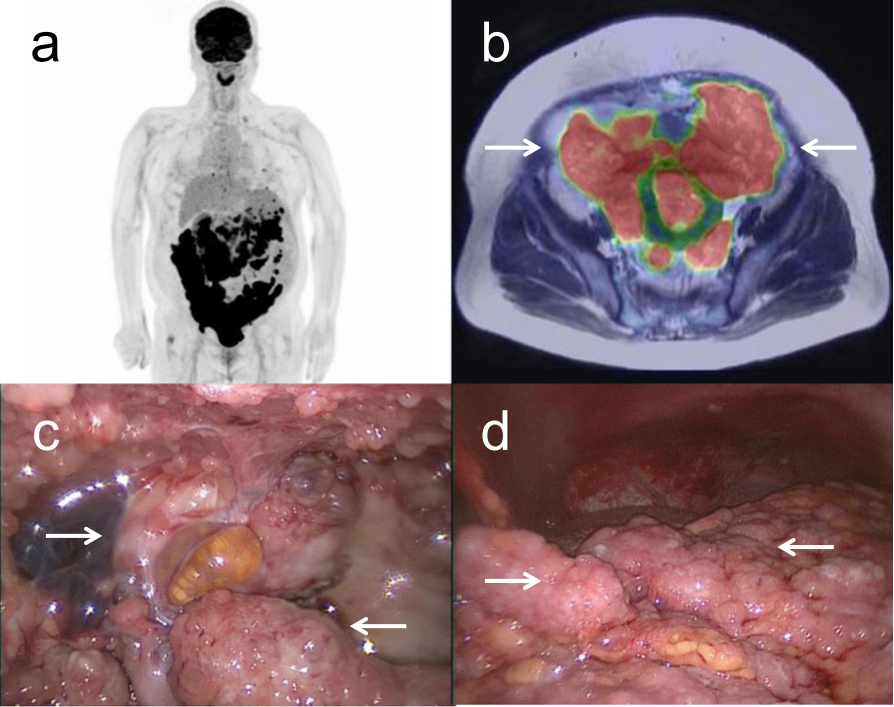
Whole-body FDG PET shows strong FDG uptake in multiple peritoneal nodule lesions (a). Integrated FDG-PET/T2-weighted MRI shows strong FDG uptake in the huge mass in the pelvic cavity (arrows) (b). Laparoscopic findings show the huge mass with a diameter greater than 20 cm in the pelvic cavity and strongly adhered to the adjacent organs (arrows) (c) and the countless disseminated lesions of various sizes in the intraperitoneal organs (arrows) (d)
